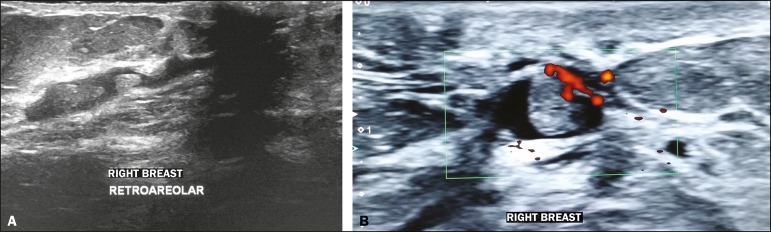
A: Ultrasound showing intraductal nodules.
